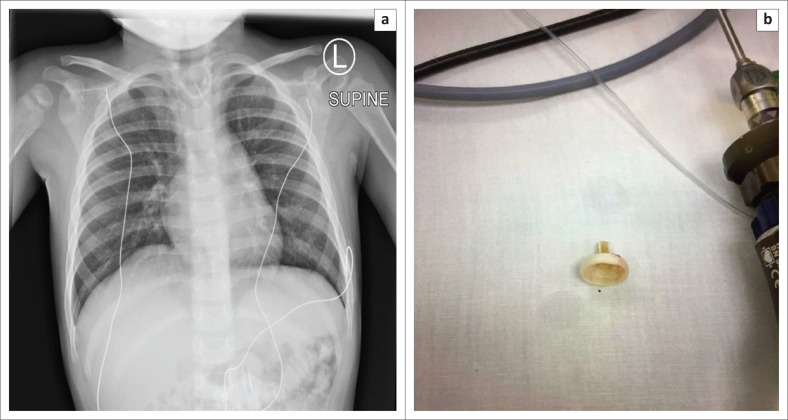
Chest radiograph (a) of a 4-year-old child showing a fractured and migrated gastrostomy tube (G-tube) component (b) in the cervical oesophagus. Note that the tracheal air shadow is seen separately, as the migrated G-tube component can mimic a tracheostomy tube.
